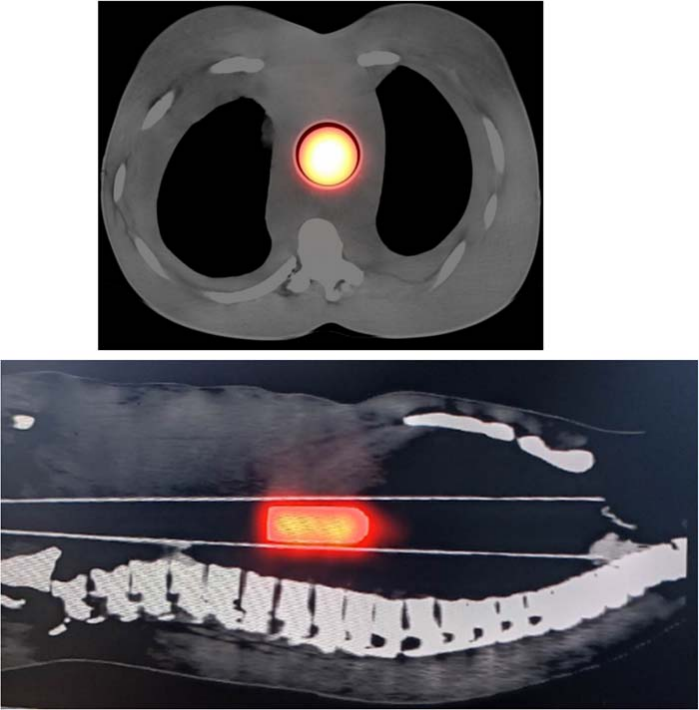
Central axial SPECT–CT slice (above) and *sagital* image of ABP with 100-mL lesion (with 4-cm diameter and 7-cm height) (below).
